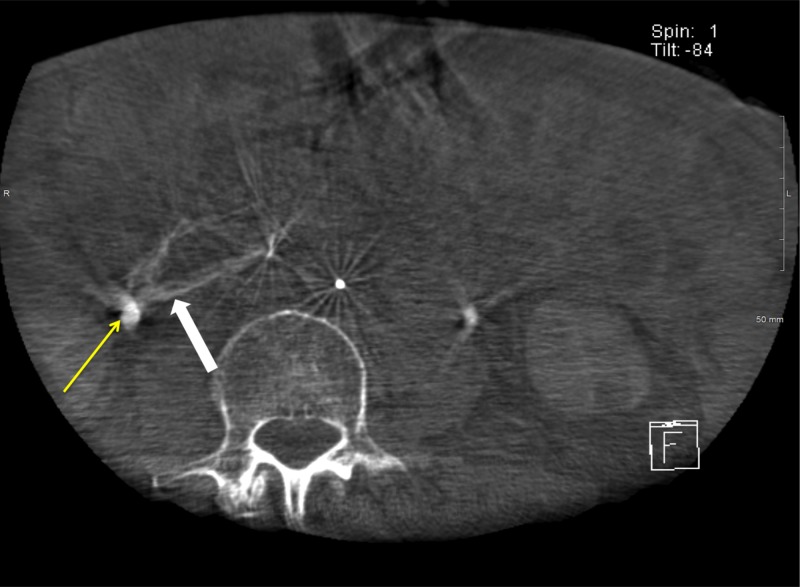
Cone beam computed tomography angiography demonstrates contrast enhancement in the duodenum. Axial cone beam computed tomography angiography of posterior inferior pancreaticoduodenal artery showing an analogous area of duodenal wall enhancement without frank extravasation (white arrow). A portion of the right ureter is also seen (yellow arrow).
